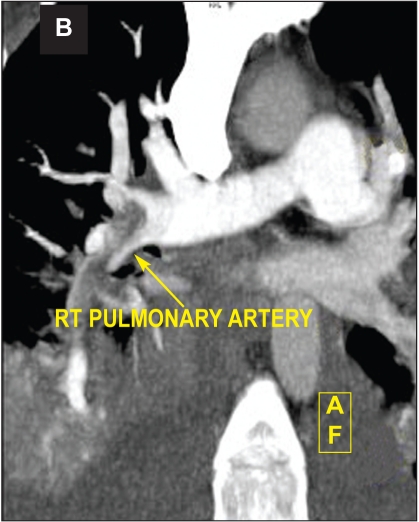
Computed tomography pulmonary angiogram showing (B, arrow) in a patient with polycythemia rubra vera, splanchnic vein thrombosis and mobile right heart thrombus.
